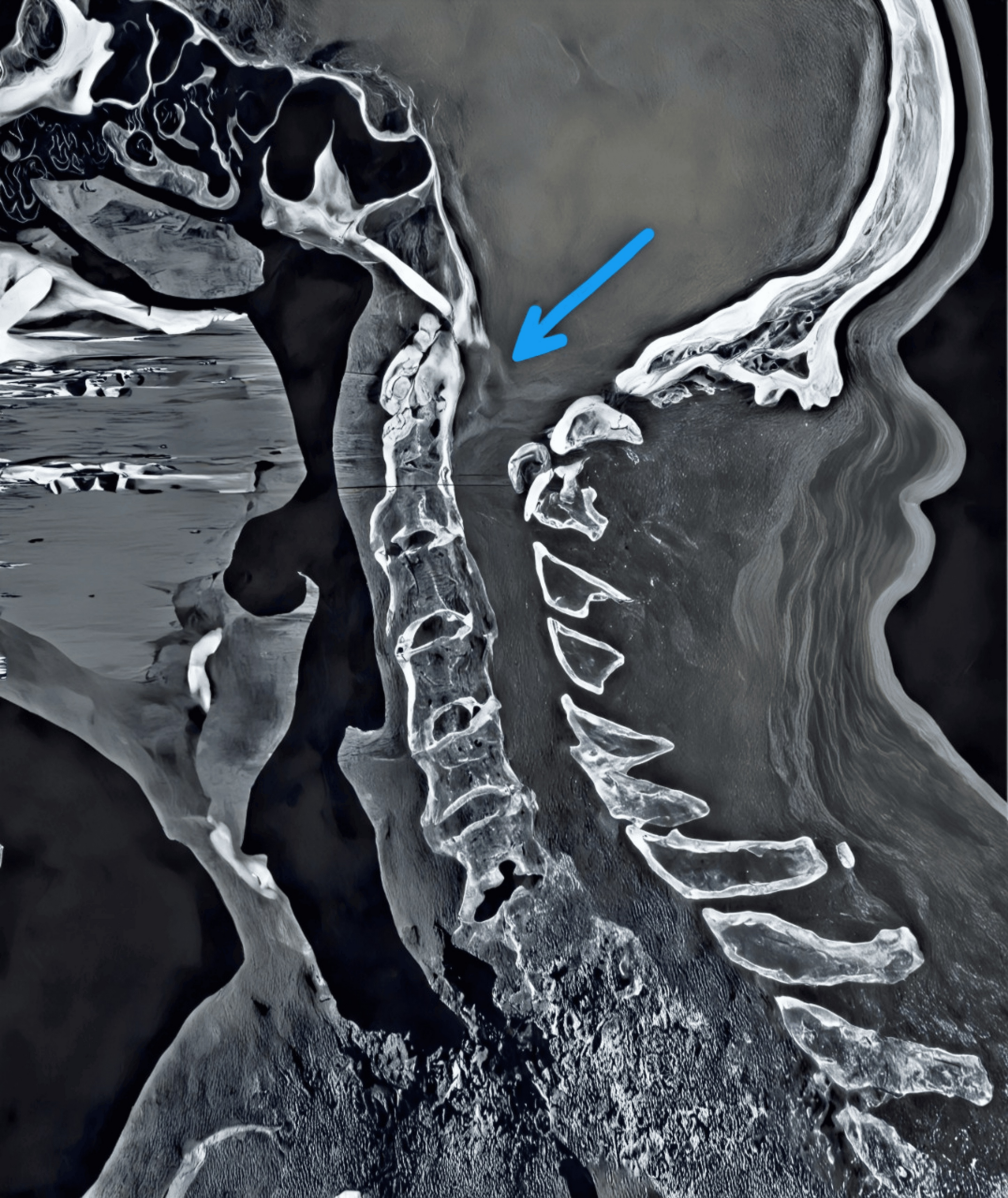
Computed tomography angiography of the cervical spine Midsagittal plane demonstrating the presence of a calcified compressive mass located in the retrodental region.
